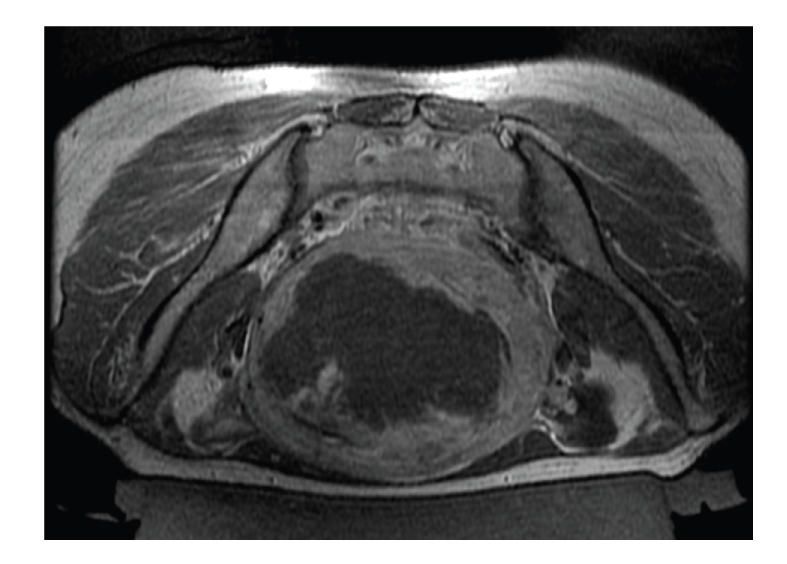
Contrast-enhanced axial T1-weighted MR image after treatment, showing nonperfused volume of 60%.
